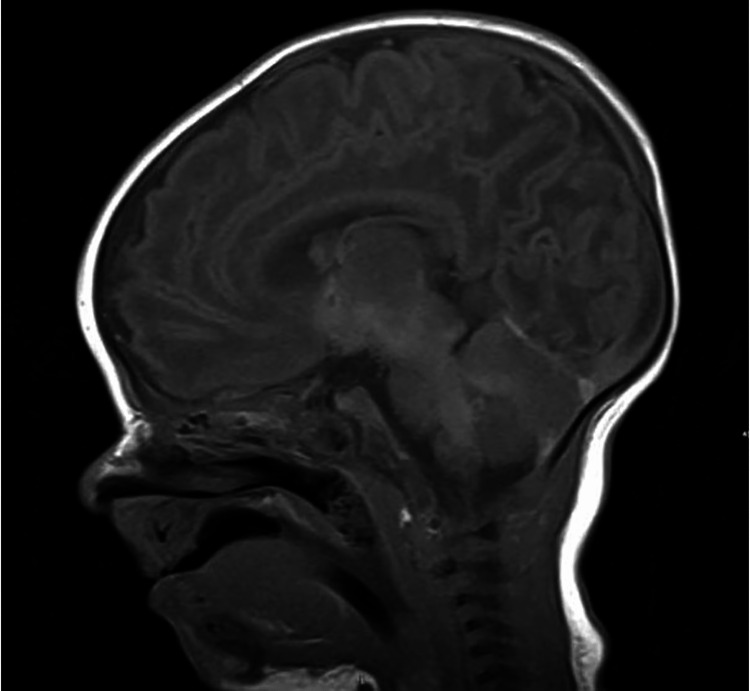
Normal MRI brain of case 2
